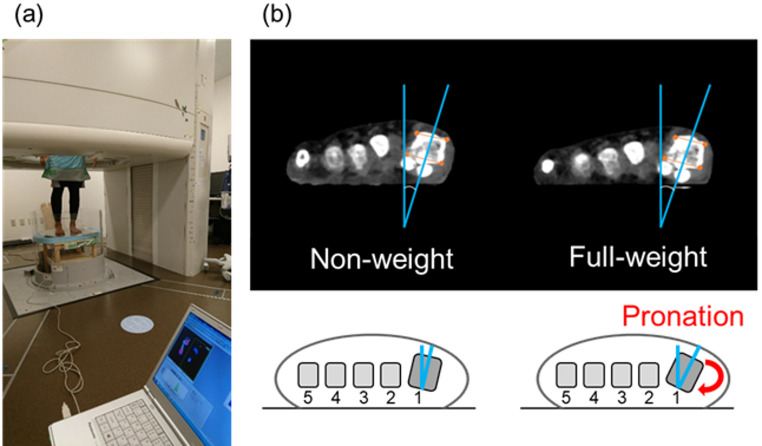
Quantification of first-metatarsal head pronation under weight-bearing. a Upright CT scans performed with plantar pressure monitoring to confirm load distribution during single-leg and bilateral weight-bearing. b Coronal-plane pronation angle of the first metatarsal head, demonstrating increased pronation under weight-bearing
